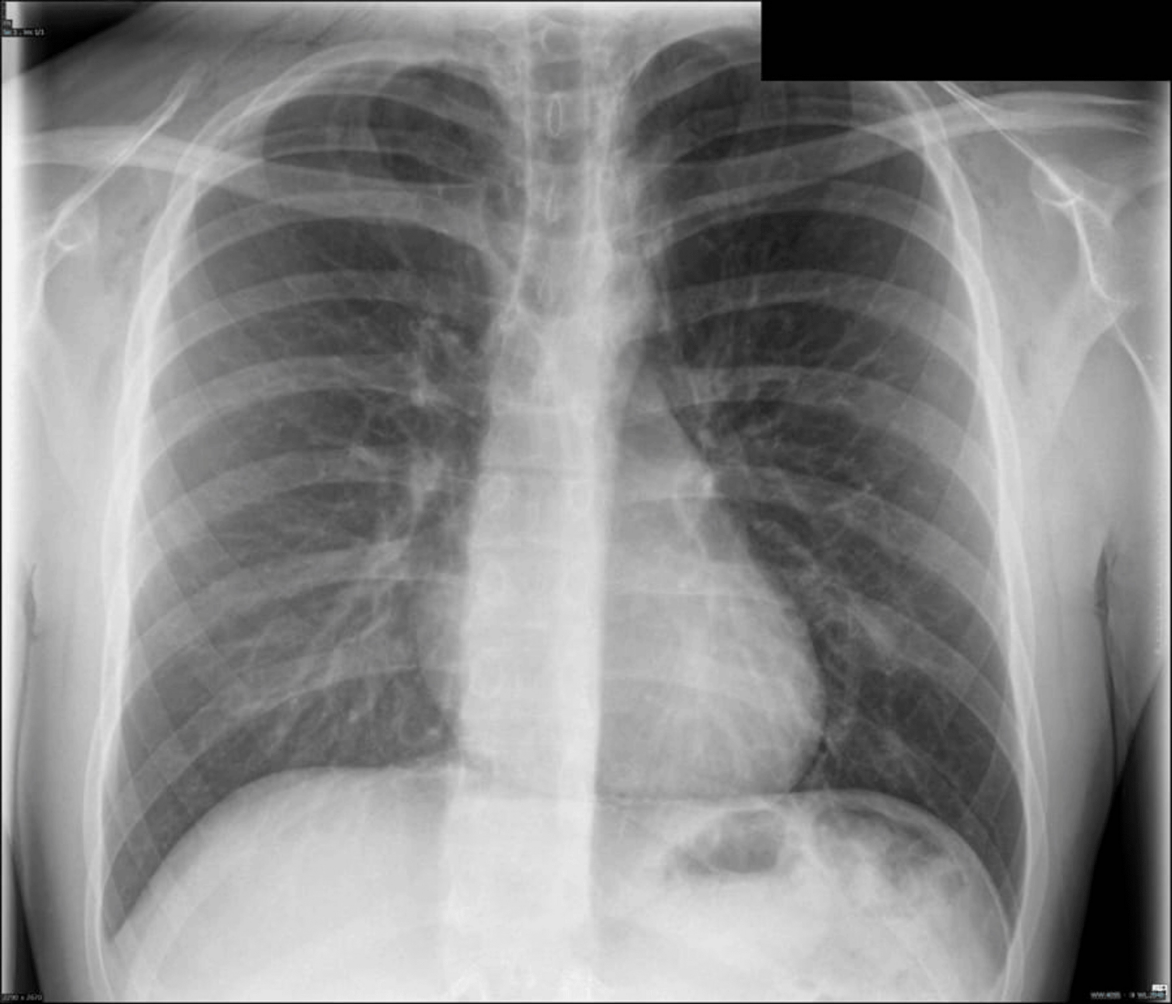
Subcutaneous emphysema in the lower neck and shoulders, with radiographic features of pneumomediastinum on the chest X-ray.
